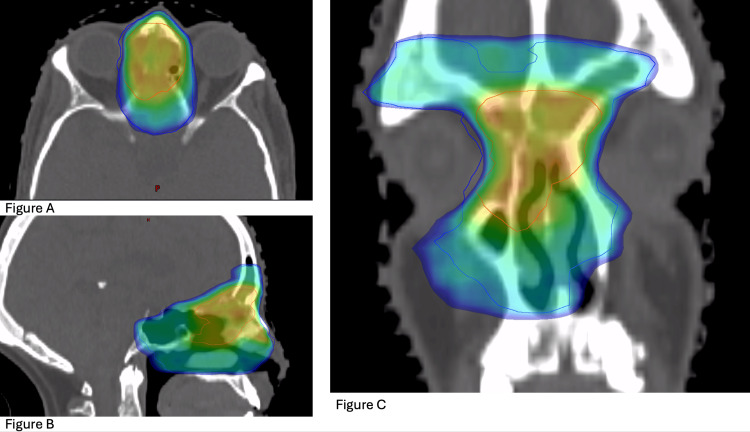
Radiotherapy target volume delineation for sinonasal undifferentiated carcinoma. Axial (A), coronal (B), and sagittal (C) contrast-enhanced CT images from radiation treatment planning demonstrate target volume contouring of the primary sinonasal mass. CT: computed tomography
